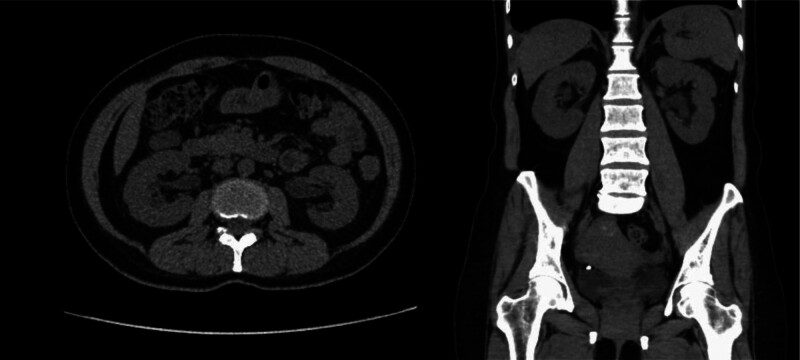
Postoperative computed tomography images showed no significant residual stones after the ureteral stent was removed.
